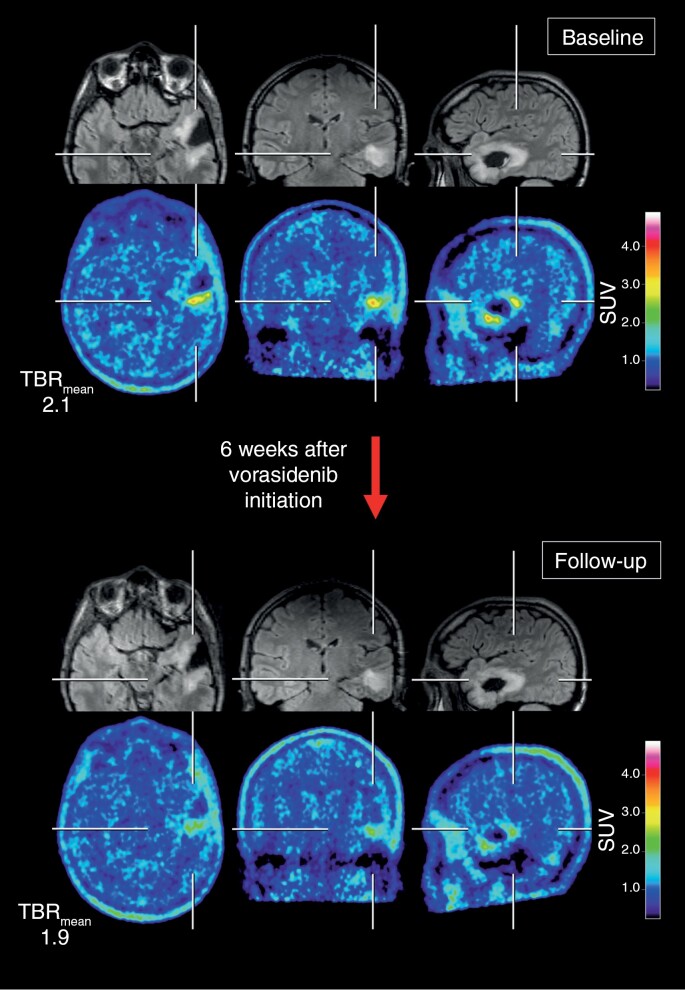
FLAIR MR and FET PET images of a 33-year-old male patient (patient #1) with a newly diagnosed and nonenhancing CNS WHO grade 3 oligodendroglioma at baseline (top rows) and at follow-up 6 weeks after vorasidenib initiation (bottom rows). After partial resection, the patient underwent vorasidenib therapy. In contrast to the unchanged hyperintense FLAIR signal, the metabolic activity decreased by 10% (ie, relative change in mean tumor-to-brain ratios from 2.1 to 1.9) indicating PET-based *Partial Response* according to the PET RANO 1.0 criteria. FET, *O*-(2-[^18^F]-fluoroethyl)-L-tyrosine.
